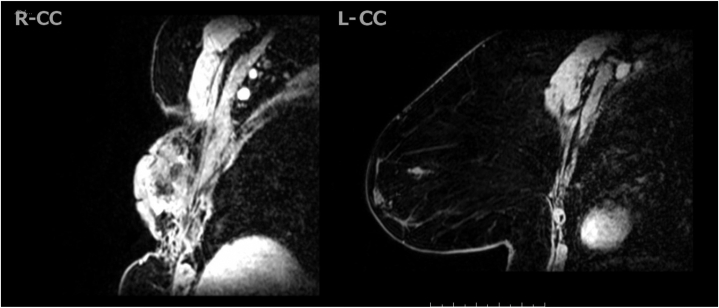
MRI of the right breast reveals contraction, skin thickening, significant abnormal enhancement, and area of probable internal necrosis spanning approximately 7.4 cm. Enhancement of the right breast extends from the skin surface to the pectoralis muscle and laterally toward the latissimus dorsi. The left breast appears unremarkable. *MRI*, Magnetic resonance imaging.
